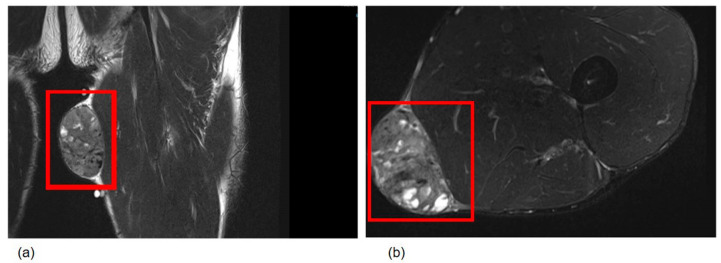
Magnetic resonance image of the left thigh, showing a well-circumscribed, solid subcutaneous mass measuring 7.0 × 5.5 × 4.0 cm with homogeneous signal intensity and no invasion into adjacent structures. (a) Coronal view; (b) axial view.
